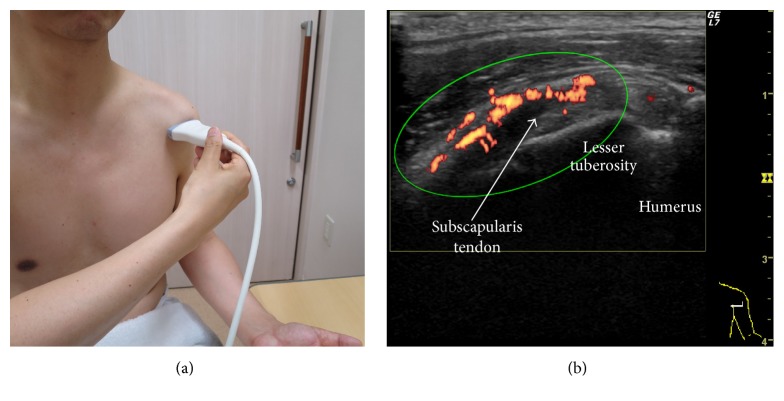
Scanning method for the evaluation of hyperemia adjacent to the anterior aspect of the subscapularis tendon. (a) Arm and probe position for the evaluation of the subscapularis tendon in the long axis. The shoulder is in a neutral position, the elbow is fixed to 90°, and the hand is spinated. The probe is placed perpendicular to the shoulder. (b) Long axis ultrasound view of the subscapularis tendon (arrow). The subscapularis tendon is superficial to the lesser tuberosity and medial to the bicipital groove. Note that the hypoechoic appearance of the medial part of the subscapularis tendon is due to the anisotropy. The area indicated by an ellipse, including the anterior soft tissues and the posterior tendon tissues and excluding the bicipital groove, is assessed for the severity of hyperemia.
